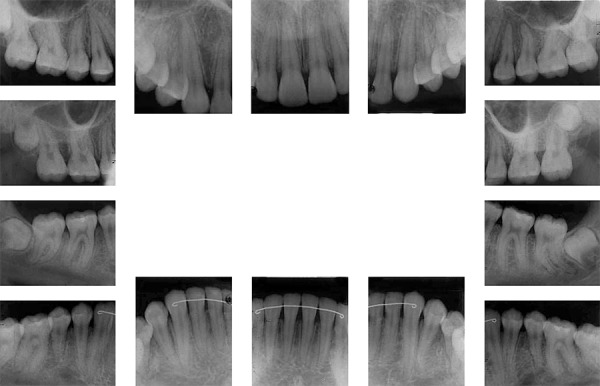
Final periapical radiographs.
